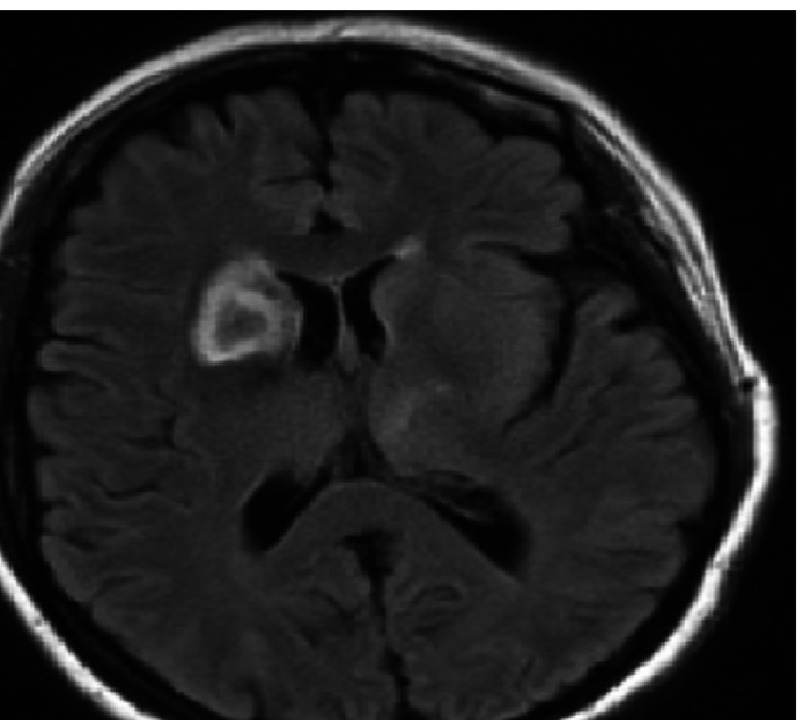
MRI brain showing hyperattenuation in the right caudate nucleus head and right lentiform nucleus An area of hypoattenuation can be also seen in the caudate nucleus head. MRI: magnetic resonance imaging
